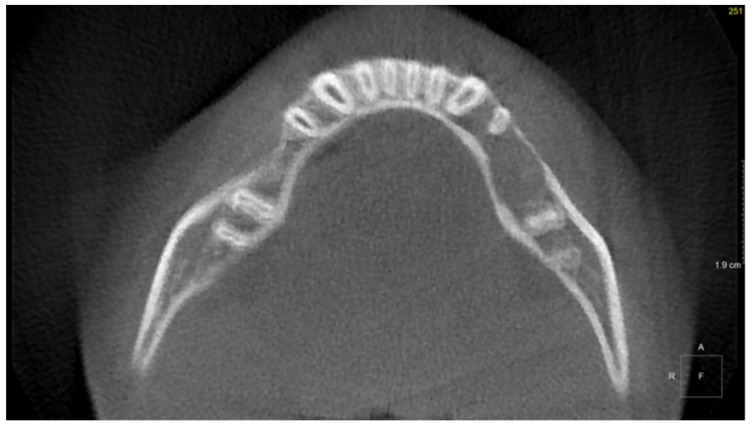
Patient, 11 y.o.: Axial image from CBCT examination with loss of trabecular pattern and thinning of cortical lingual and buccal plates in the region of teeth 33, 34, and 35.
